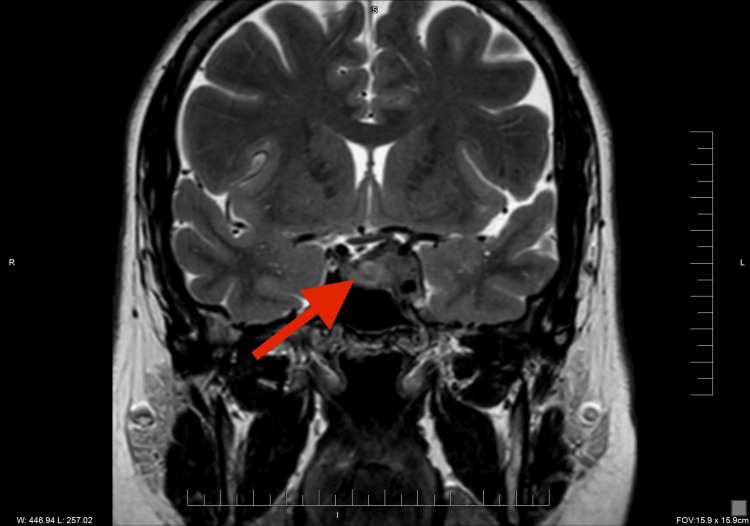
Coronal T2-weighted MRI Coronal view confirms asymmetric invasion of the left cavernous sinus (red arrow) and lateral displacement of the pituitary stalk; the optic chiasm is mildly compressed superiorly.
